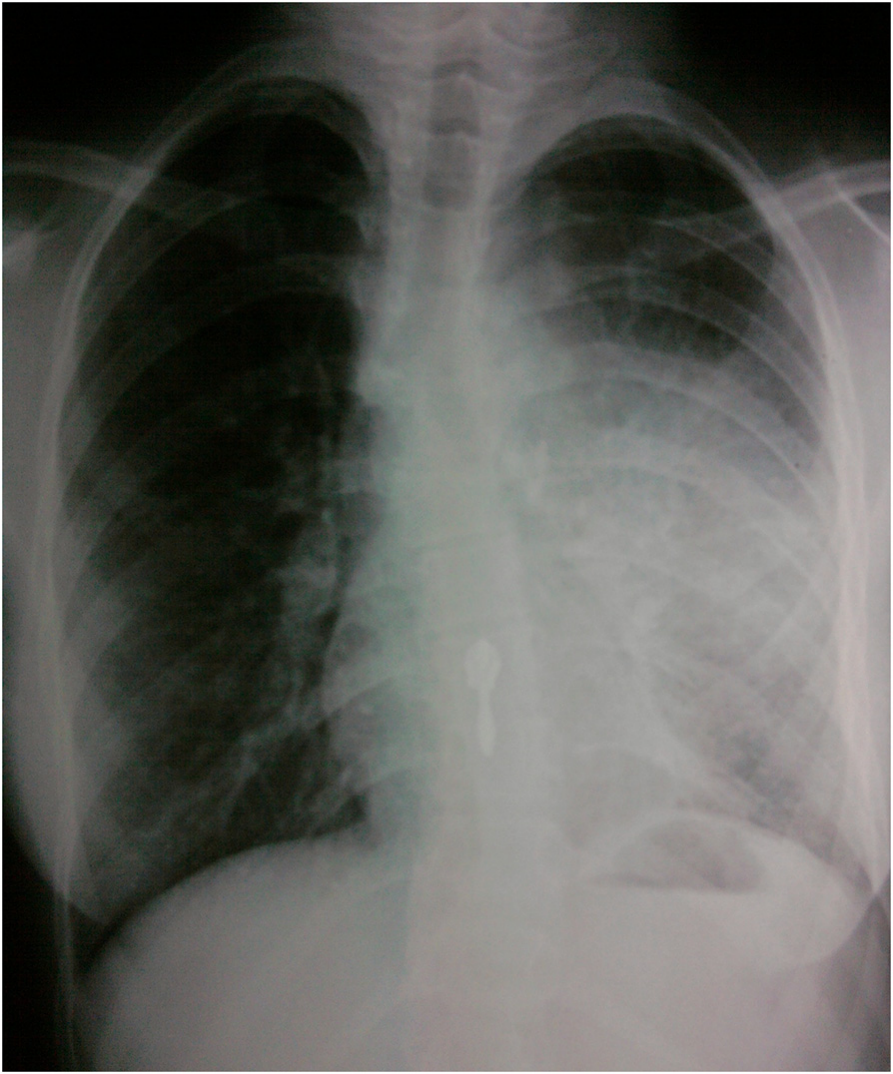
Chest radiography: left anterior mediastinal tumor.
